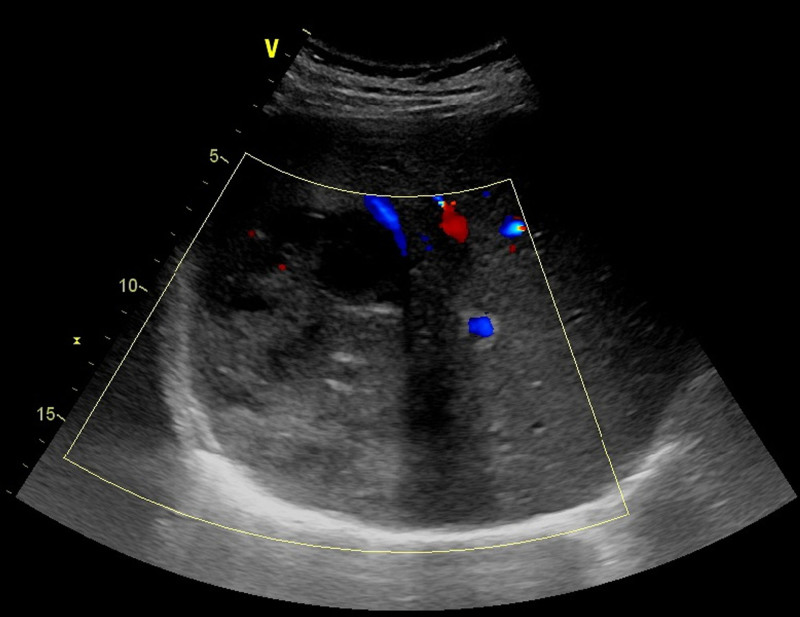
Ultrasonography images of the hepatic inflammatory myofibroblastic tumor.
